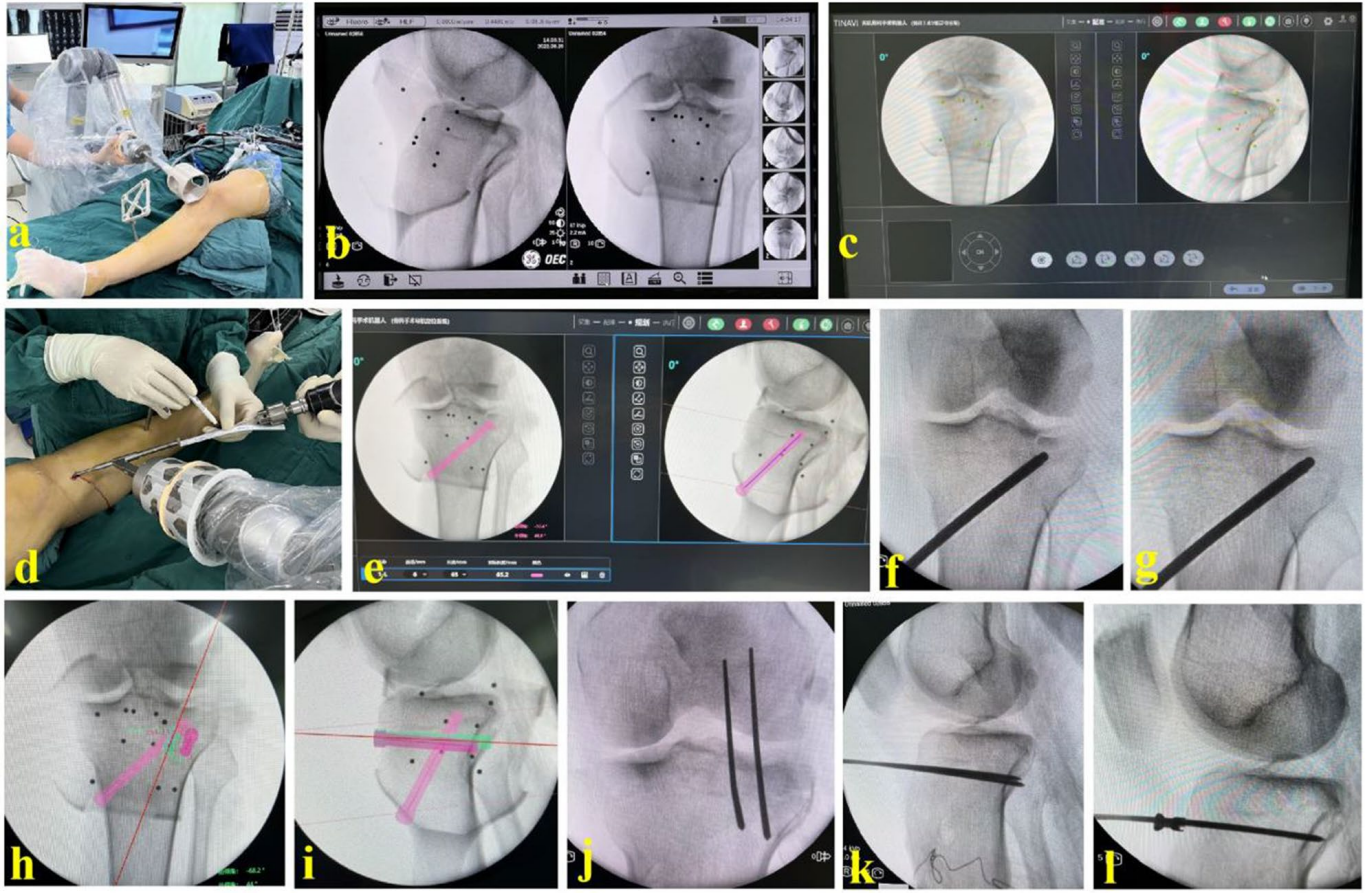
The intraoperative setting up and application procedures of the orthopedic robot system for the PL-depression tibia plateau fractures. (a,b) The robot system was set up and standard AP and lateral X-ray images of the tibial plateau were achieved; (c) The standard AP and lateral X-ray images were imported into the planning and control software; (d–g) The reduction path was planned on the planning and control software, and the PL-depression fragment was successfully reduced by a reduction bone rod via robot navigation; (h–l) The absorbable screw channels were planned on the planning and control software, and two absorbable screws were inserted via robot navigation.
